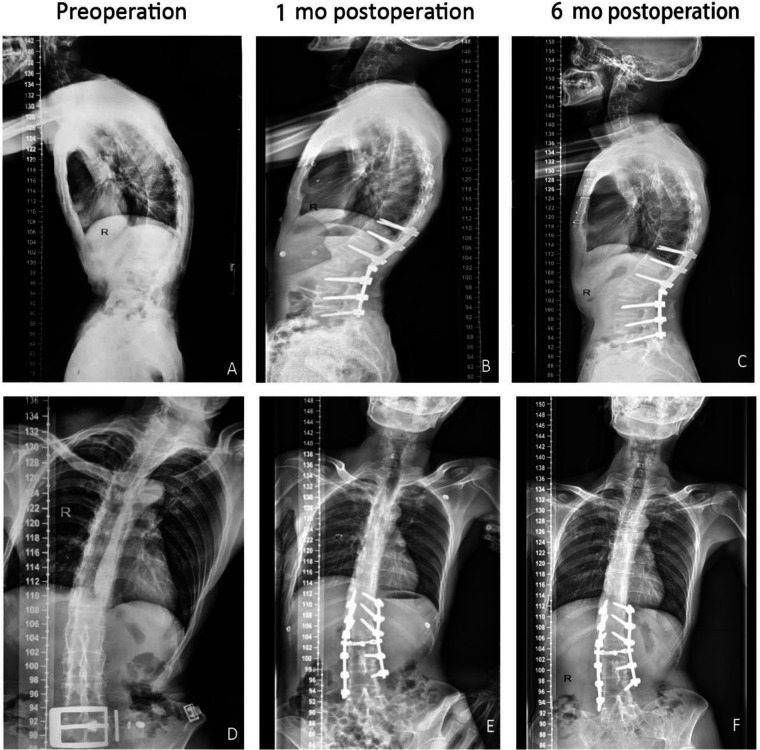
Male, aged 40, ankylosing spondylitis with kyphosis, preoperative whole spine lateral radiographs showed thoracolumbar kyphosis, spine sagittal imbalance (A), cobb angle 40° (D). The spine sagittal rebalanced (B,C) and the Cobb angle corrected to 20° (E,F), tested 1 month and 6 months after operation, respectively.
